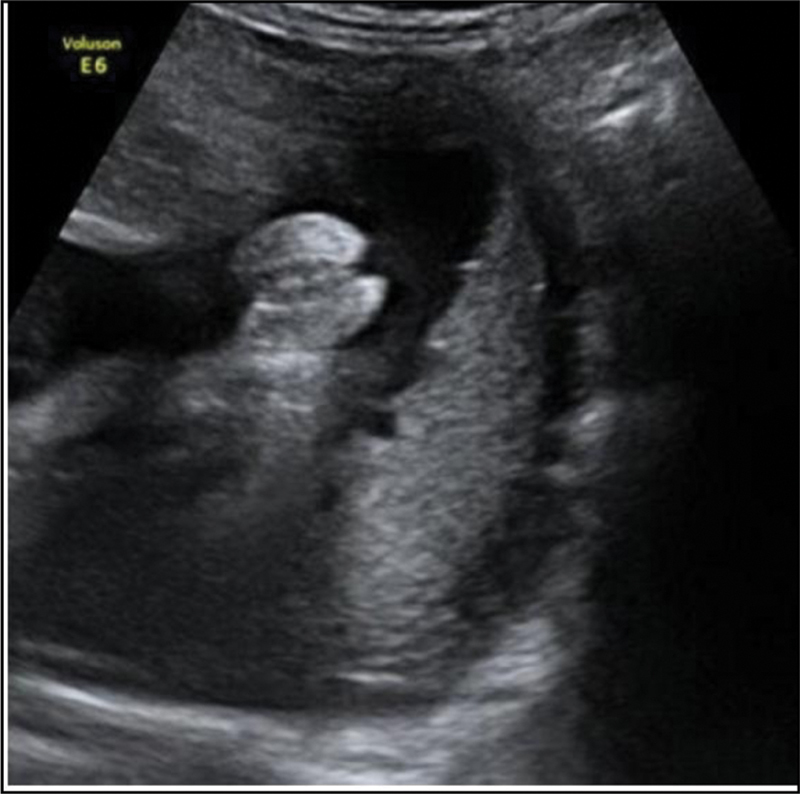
Identification of female external genitalia on the midtrimester ultrasound scan.
